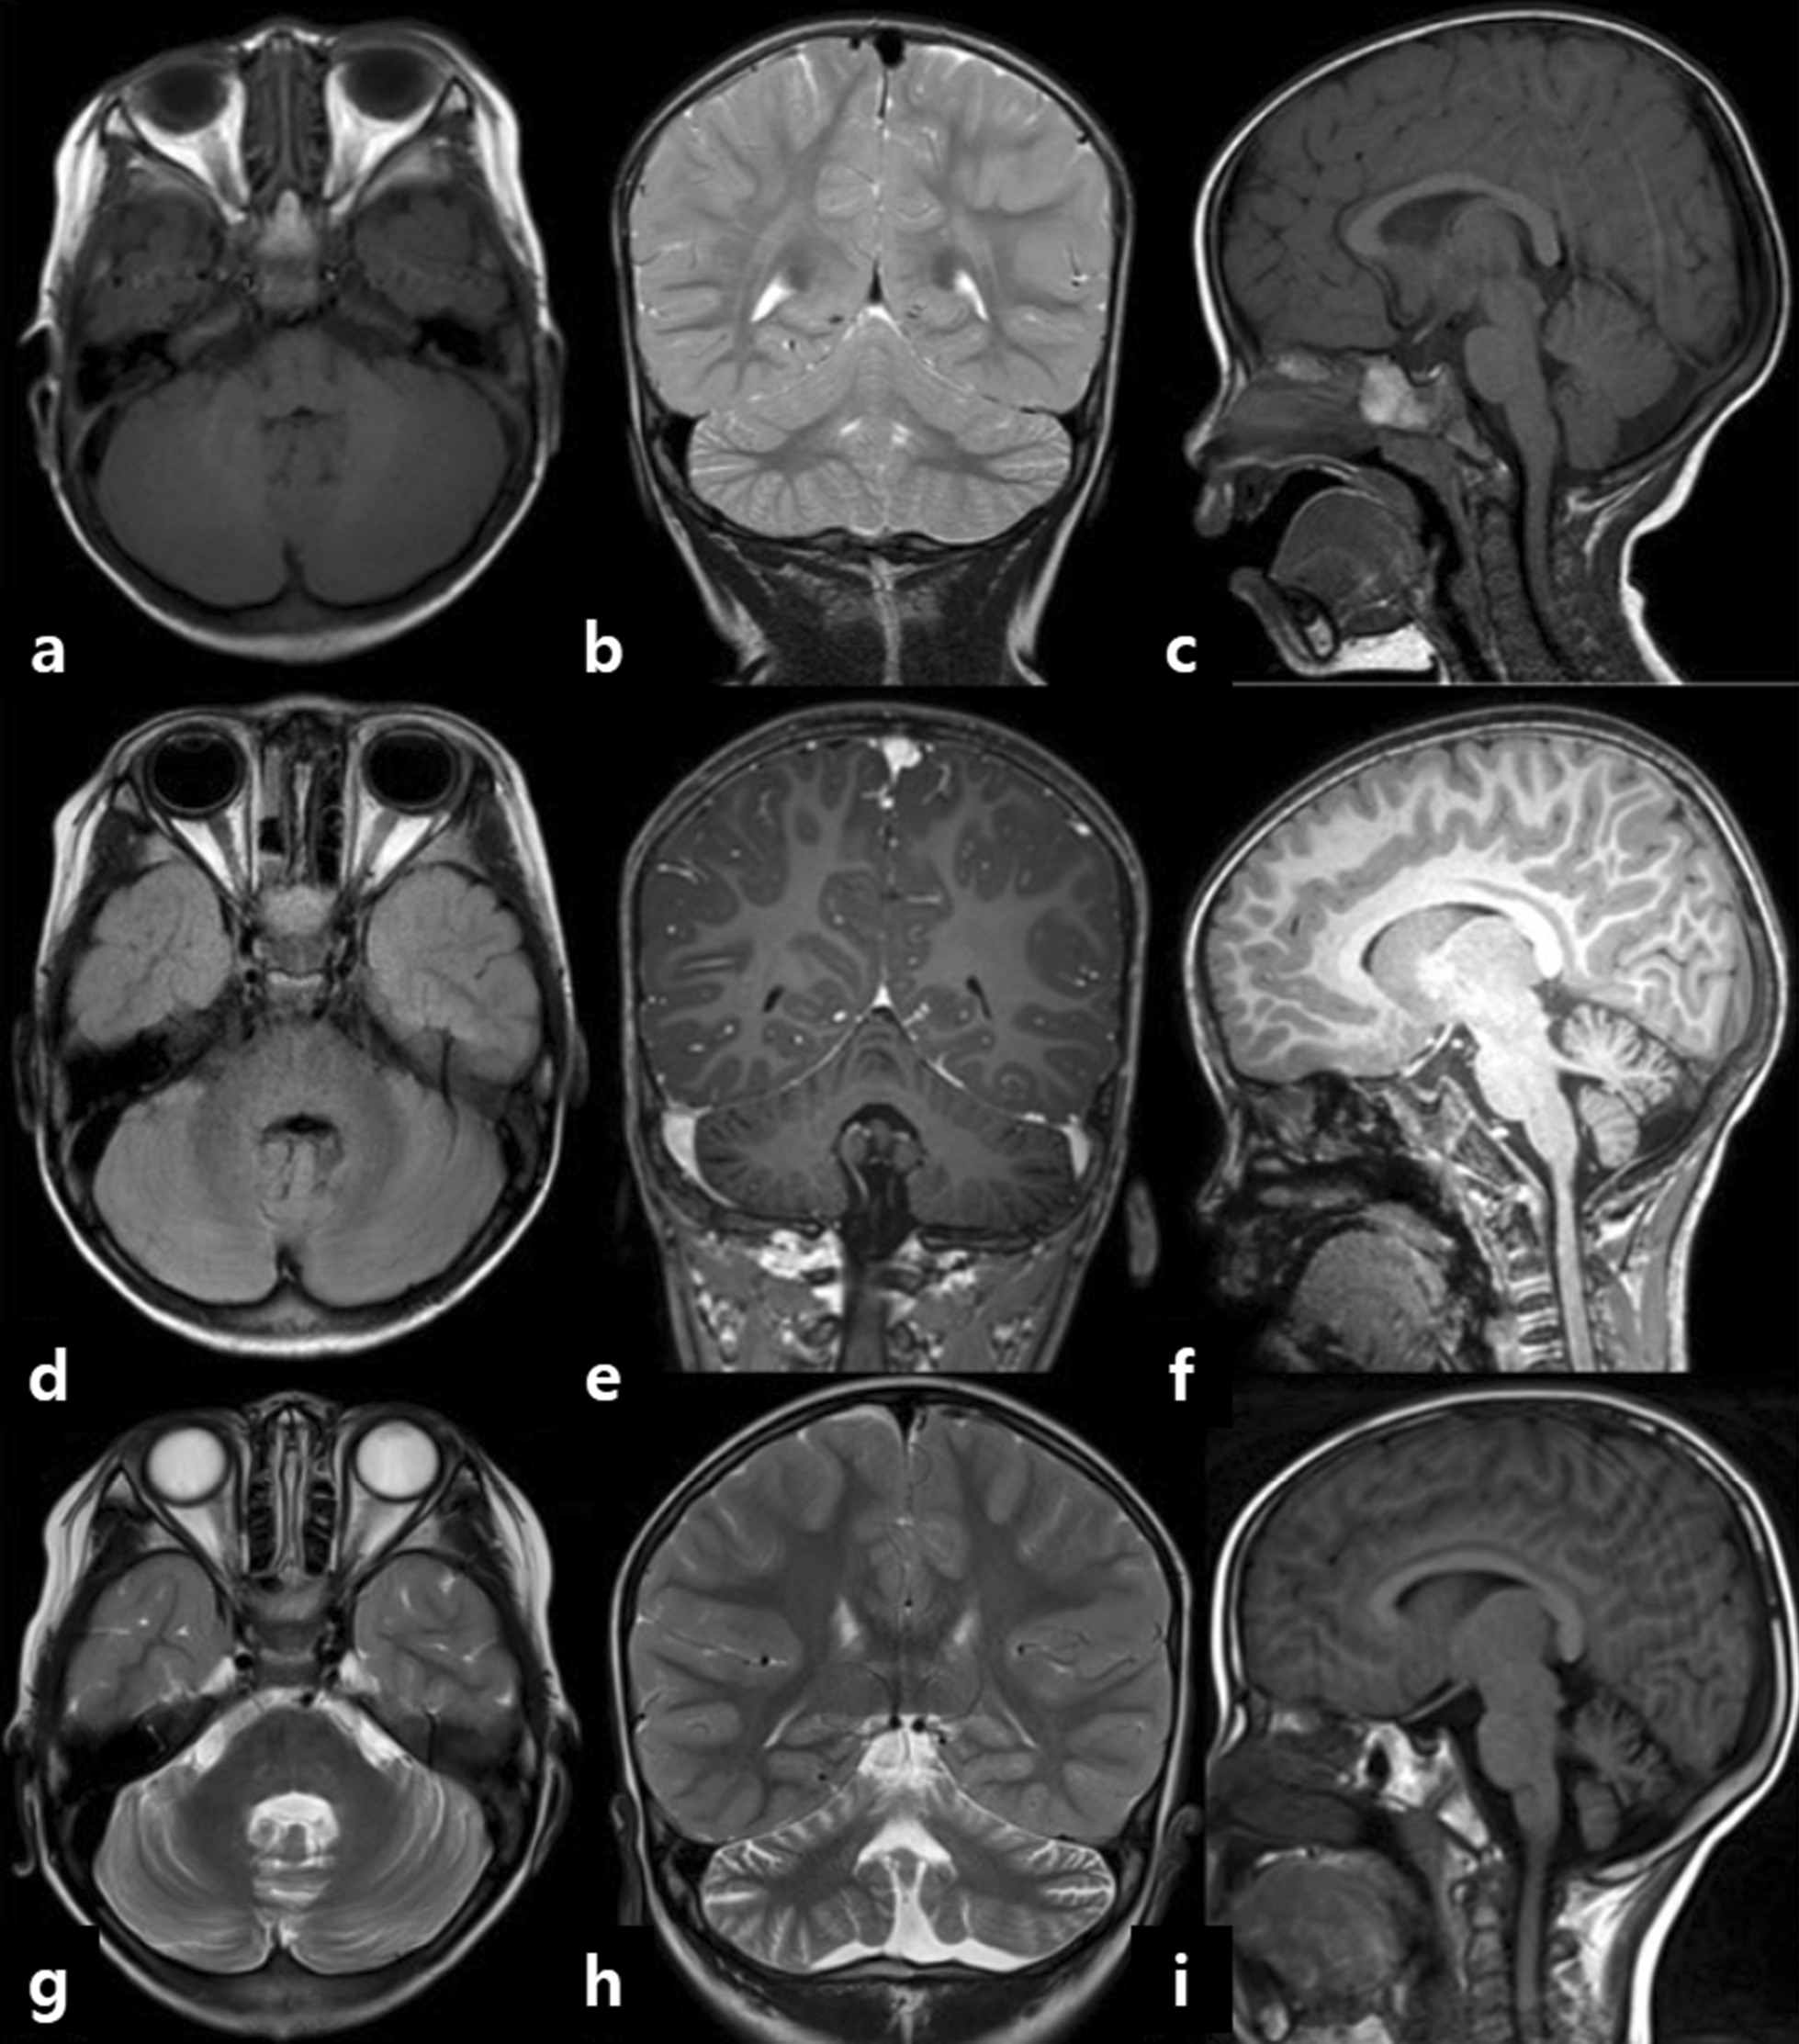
Brain MRI of the patient at 1 year (a–c), 5 years (d–f), and 7 years (g–i). Interval development of mild atrophy in the cerebellar vermis and hemispheres and subtle increased FLAIR signal intensity were noted. *MRI* magnetic resonance imaging, *FLAIR* fluid-attenuated inversion recovery
